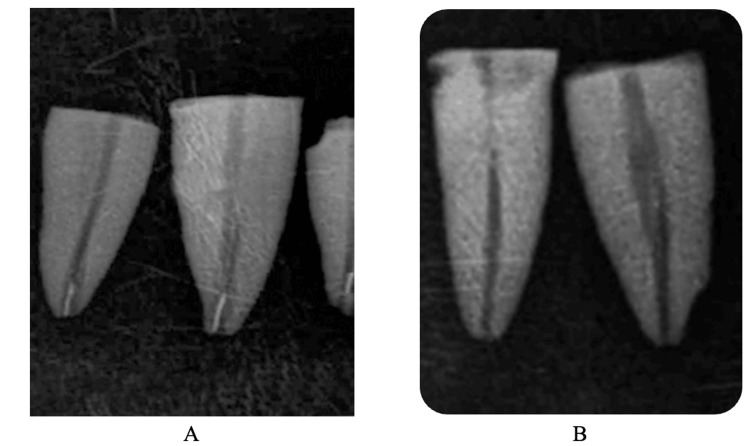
Postoperative X-rays (A) Three samples with a successful bypass of the fractured instrument, showing different remaining dimensions of the Ni-Ti fragment (B): Two samples showing a complete elimination of the broken file.
